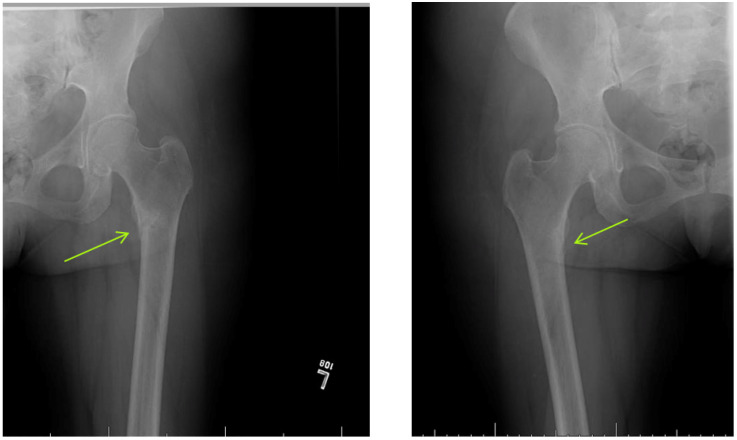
Hip/Femur X-Ray. Patchy regions of sclerosis in the medial subtrochanteric regions, Left (L) >Right (R) corresponding to uptake on bone scan concerning for subacute incomplete fractures. Consistent with Looser’s Zones.
